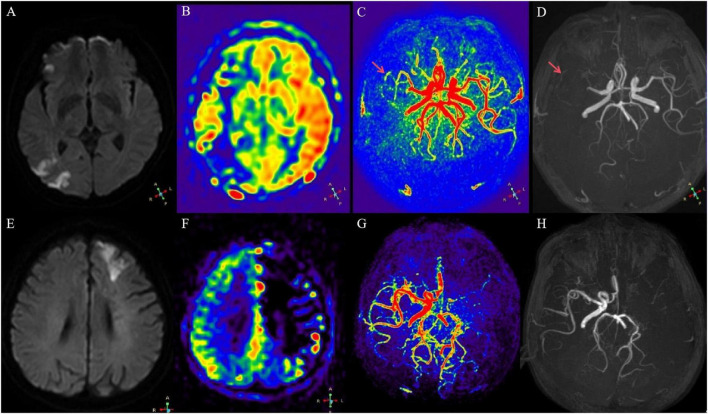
(A–D) A 48-year-old male with dizziness, right limb weakness, and sensory impairment. (A) Multiple and limited diffusion in the right frontal parietal lobe of DWI. (B) There was a large area of hypoperfusion around 3D-ASL lesions. (C) At the peak of vascular perfusion, 4D-TRANCE angiography showed that the M1 segment of the middle cerebral artery was occluded, and collateral vessels were visible (arrow). (D) TOF-MRA only showed occlusion of the M1 segment of the right middle cerebral artery (arrow). (E–H) A 49-year-old male with right lower limb weakness. (E) DWI showed limited left frontal lobe diffusion. (F) There was a large hypoperfusion area around 3D-ASL lesions. (G) At the peak of vascular perfusion, 4D-TRANCE angiography showed that the M1 segment of the middle cerebral artery was blocked, and no collateral vessels were found. (H) TOF-MRA showed occlusion of the M1 segment of left middle cerebral artery.
